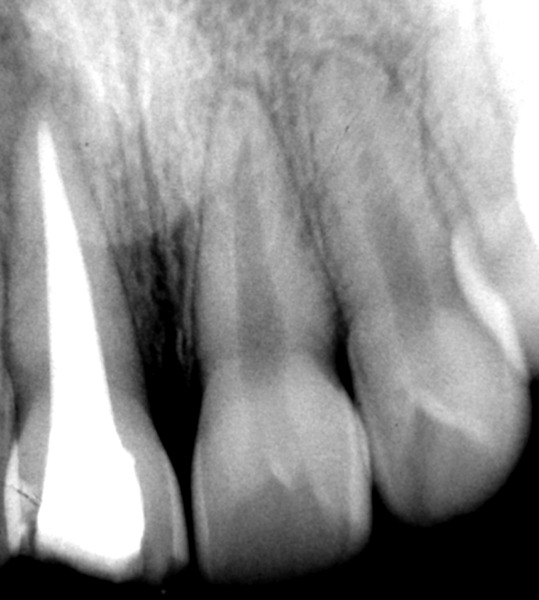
Radiograph after completion of root canal treatment
